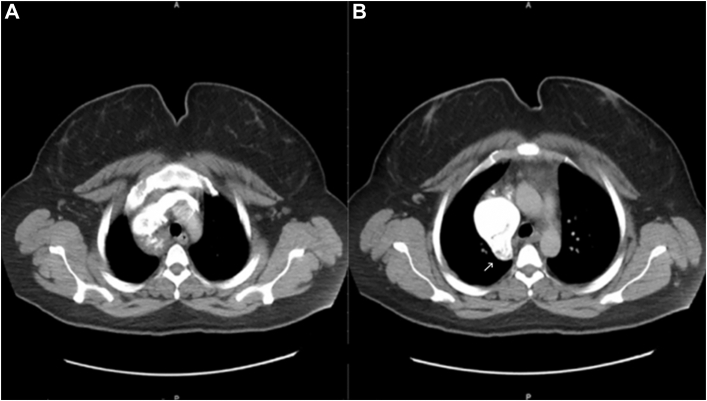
Computed tomographic angiography. Axial view showing (A) the innominate vein and (B) the azygos vein contribution to the superior vena cava saccular aneurysm (arrow).
